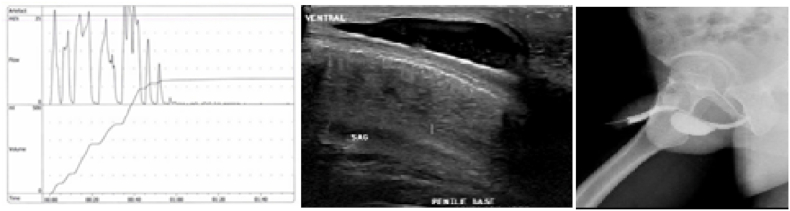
Preoperative investigations; Uroflowmetry, ultrasound and Urethrogram.
